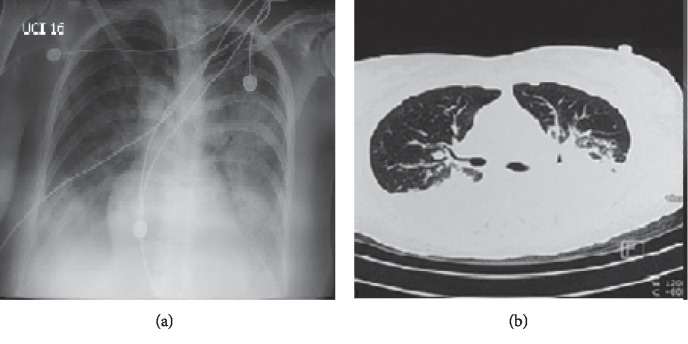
(a) Chest X-ray; AP view: bilateral, diffuse basal and perihilar alveolar infiltrate; (b) chest CT: bilateral effusion, increased vascularity, with bilateral infiltrates that converges to the posterior area.
